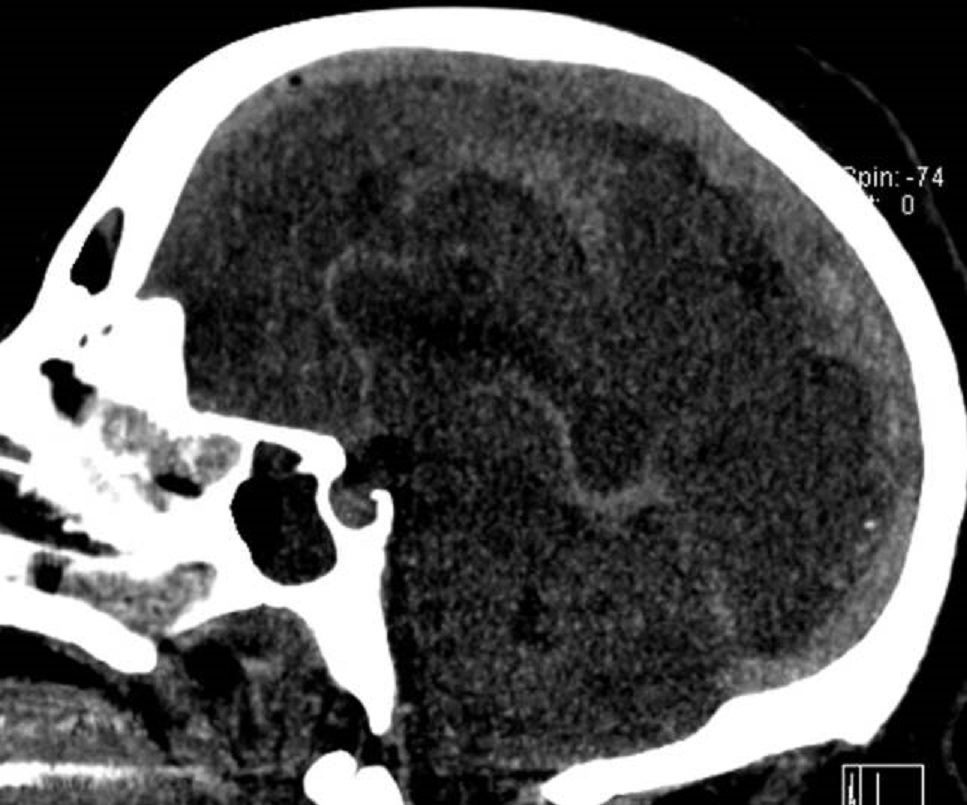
CT Venogram Post Second Infusion CT venogram obtained after second infusion with larger catheter, increased tPA dose, and larger volume of infusion reveals flow through sagittal sinus and flow through internal cerebral veins. CT also reveals air resulting from infusion.
